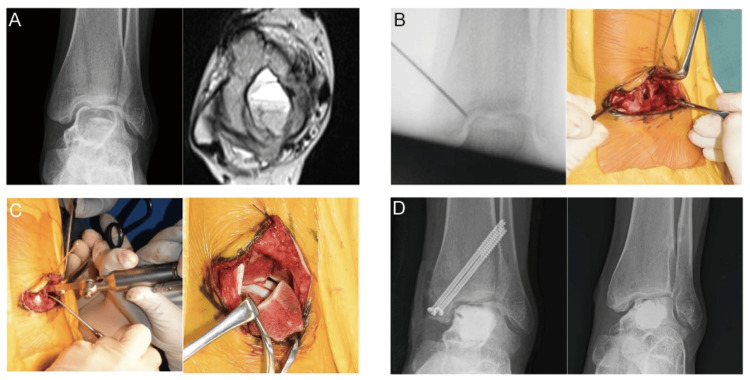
Case 2: pre- and postoperative images and intraoperative photographs. (A) (Left) Radiograph demonstrates an osteolytic lesion in the talar body, and preoperative osteoarthritic change is already evident. (Right) MRI depicts a bone tumor with a fluid-fluid level, respectively. (B) Intraoperative findings: the left image fluoroscopically confirms the apex of the reverse‑V chevron osteotomy, whereas the right image shows a U-shaped skin incision with dissection extended through the subcutaneous and fascial layers. (C) Osteotomy and talar exposure: the left image depicts the performance of the chevron-type medial malleolar osteotomy, whereas the right image shows the preservation of the deltoid ligament and the distal reflection of the osteotomized fragment for talar exposure. (D) Postoperative radiographs: the left image was obtained immediately after surgery, whereas the right image, taken at the 39-month follow-up, demonstrates no evidence of recurrence despite residual osteoarthritic change and the collapse of the medial talar dome. MRI: magnetic resonance imaging
